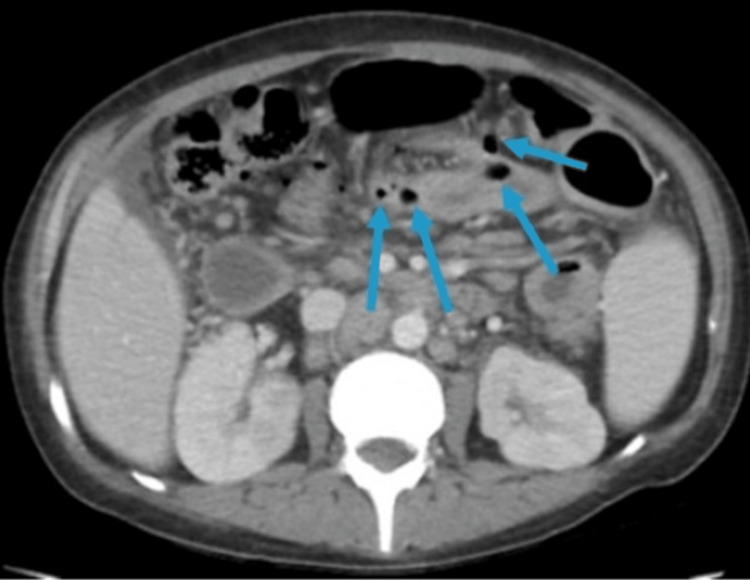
Axial contrast-enhanced CT scan showing severe small bowel enteritis with microperforations (indicated by arrows)
